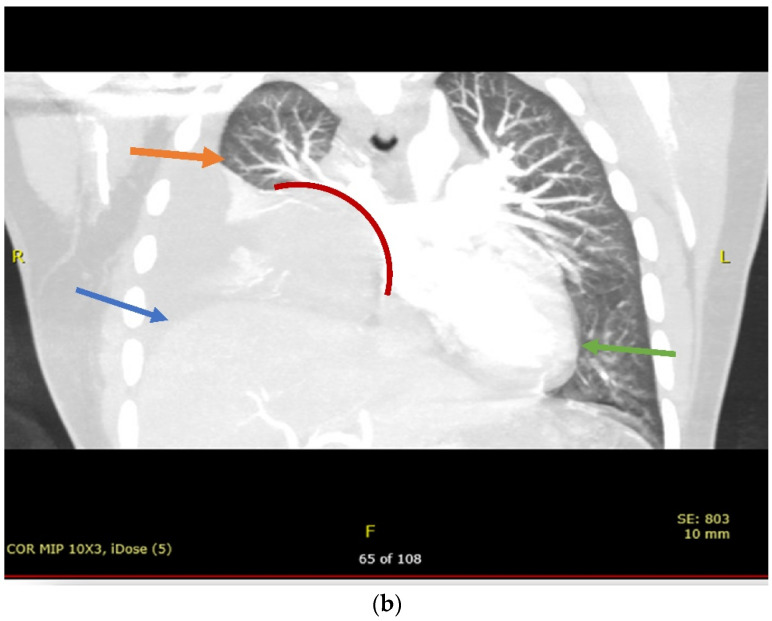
(a) Axial view of chest CTA showing mediastinal mass (blue arrows) compressing the right atria and ventricle along with right lung atelectasis and cardiac displacement to the left. Right sided pleural effusion (yellow arrow) can also be appreciated in the image. (b) Coronal view of chest CTA showing mediastinal mass compressing the right atria and right ventricle (red margin) along with right lung atelectasis (yellow arrow) and cardiac displacement to the left (green arrow). Right sided pleural effusion (blue arrow) can also be appreciated in the image.
